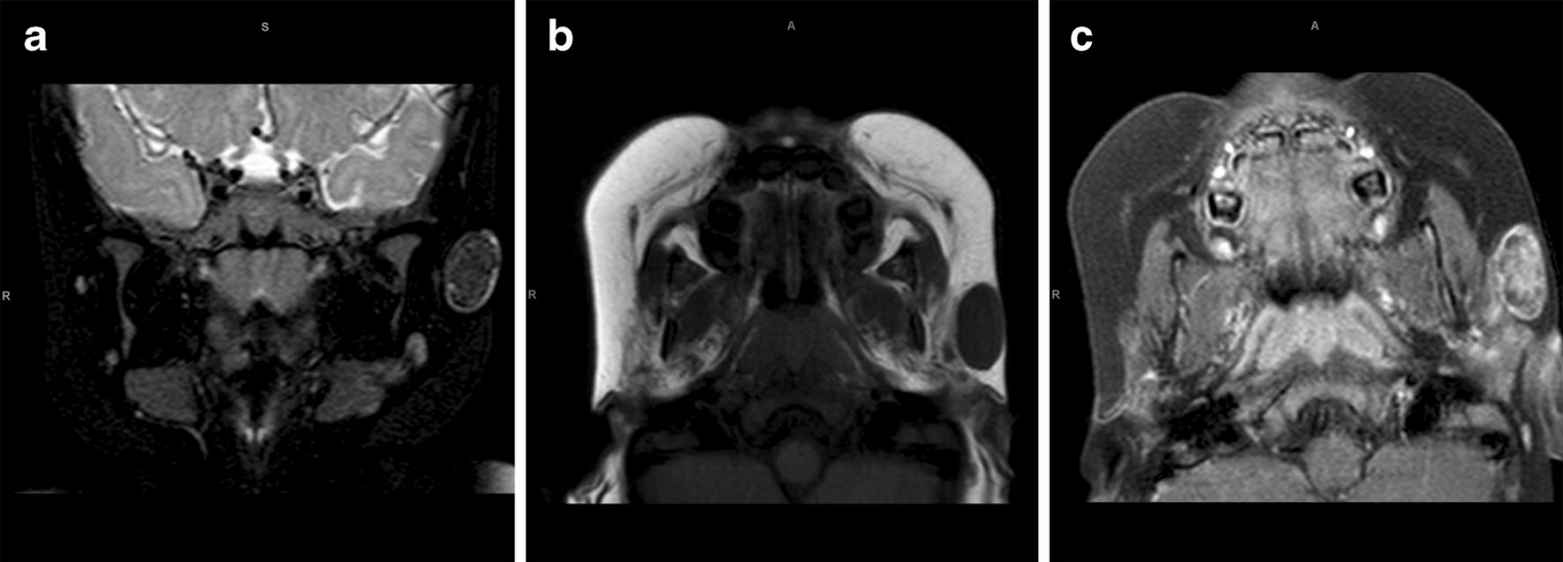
Pilomatricoma. Coronal T2-W (a) and axial T1-W (b) MRI shows a hypointense 23 × 12 mm subcutaneous nodule on both views, with a well-defined T2 hyperintense rim (a). On the T1-W post-contrast view (c), the rim enhances avidly and the central component heterogeneously
